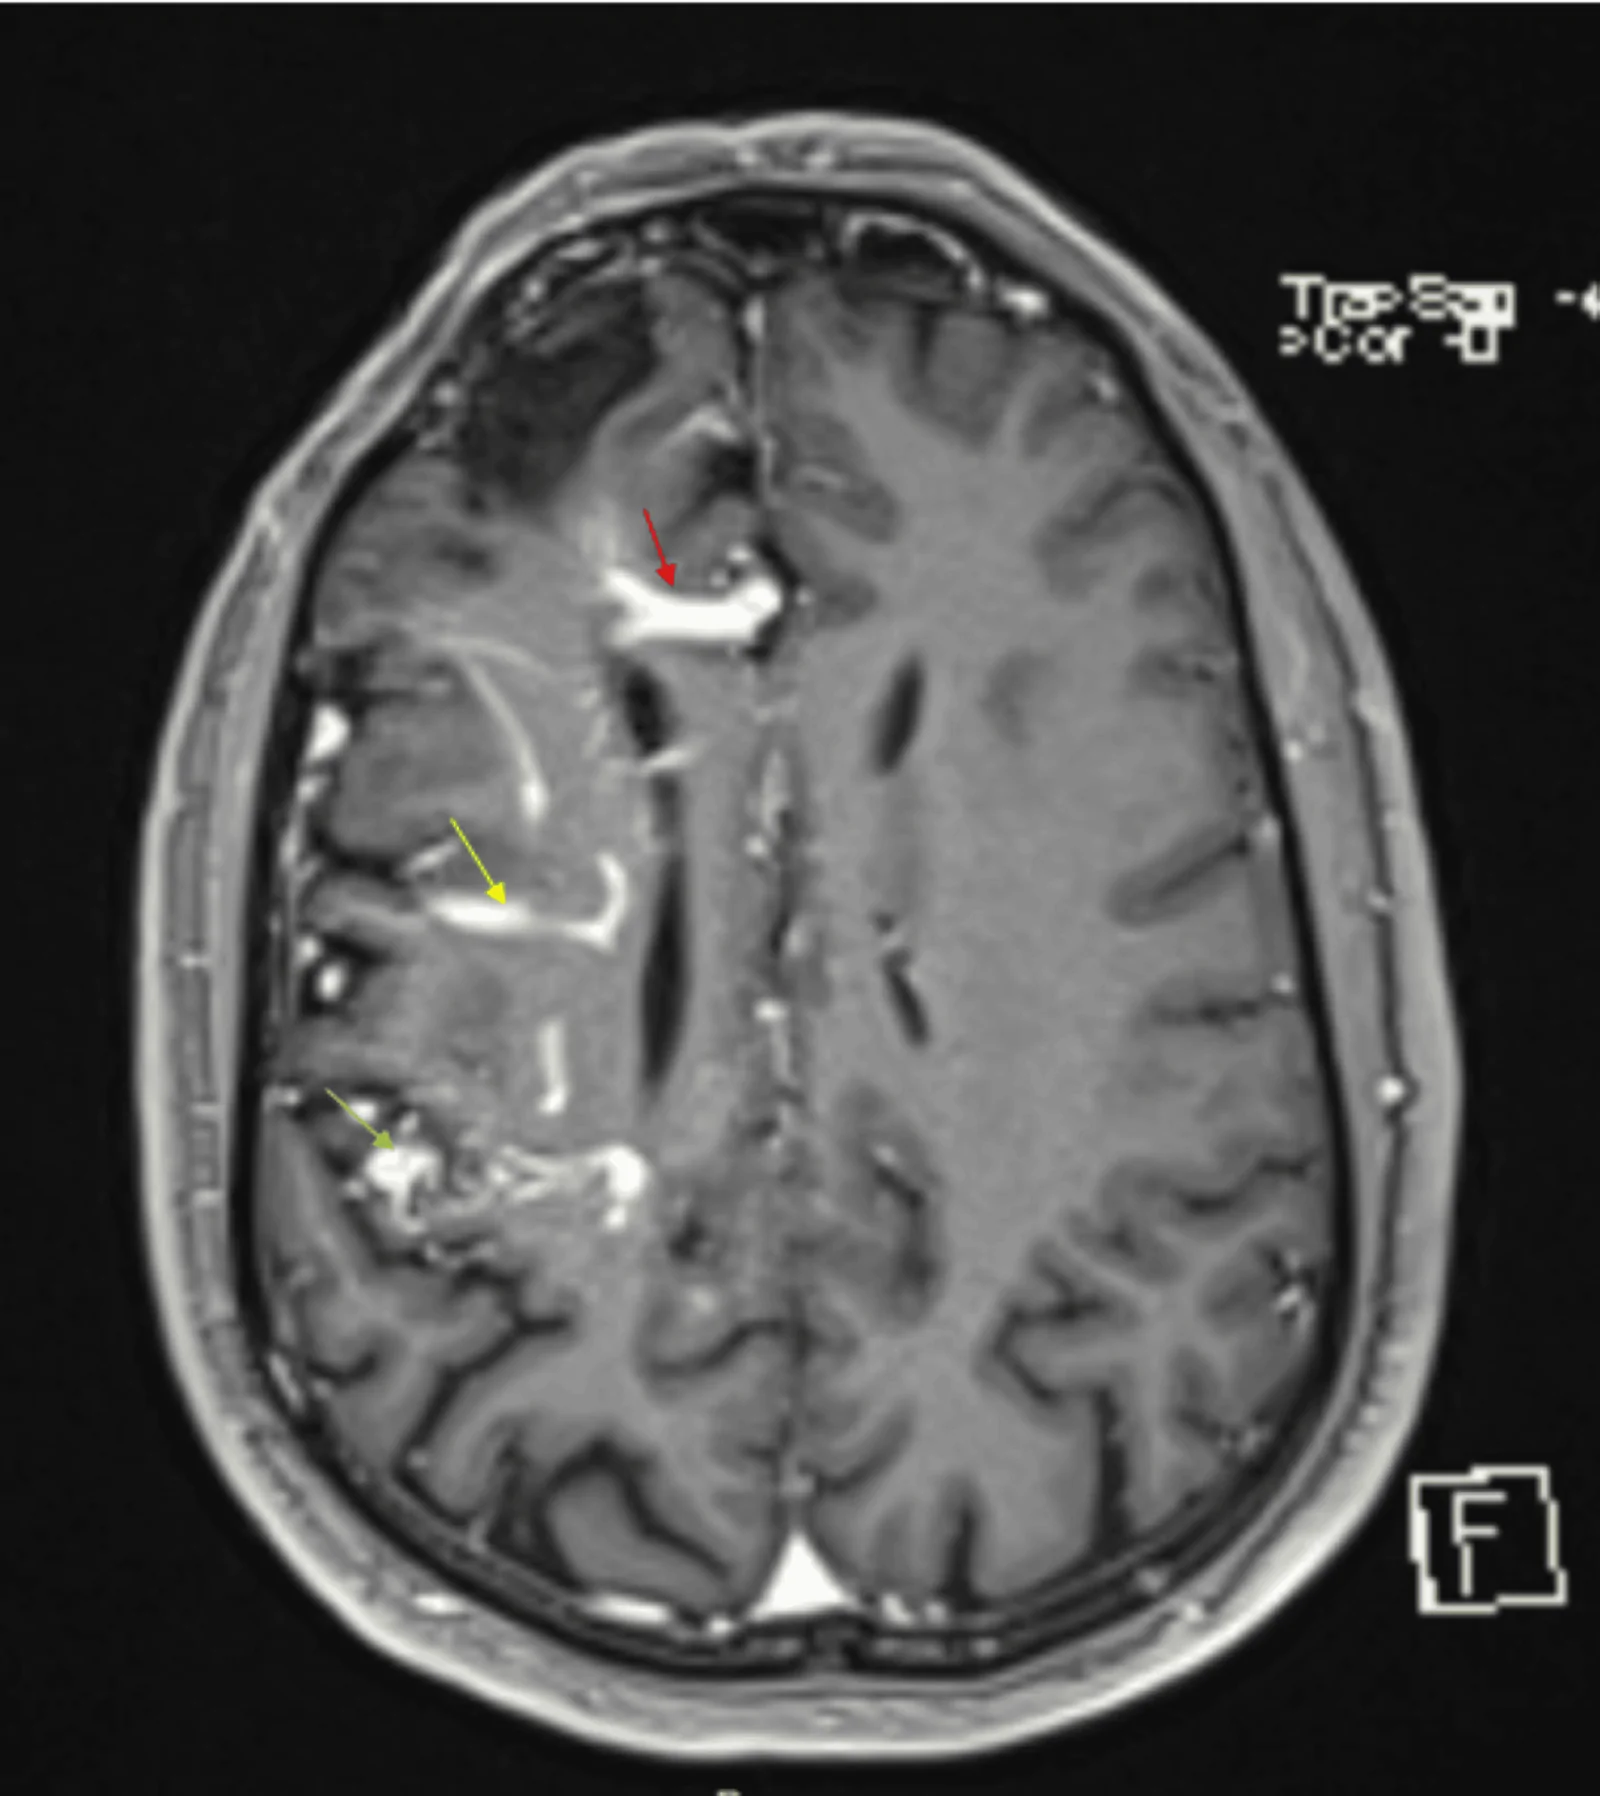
Brain MRI Post-contrast axial T1-weighted image demonstrating multiple, radially oriented medullary veins (yellow arrow) converging into a dilated draining vein (red arrow), consistent with a right hemispheric developmental venous anomaly (DVA). Note the AVM (green arrow). AVM: arteriovenous malformation
